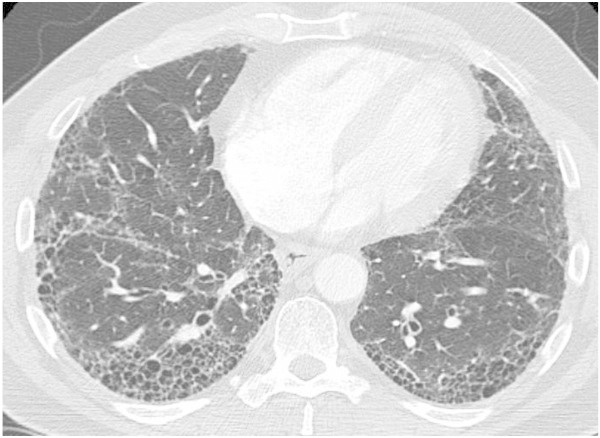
HRCT cross-sectional view showing a pattern of peripheral reticulation and honeycomb change that is diagnostic of the presence of UIP.
